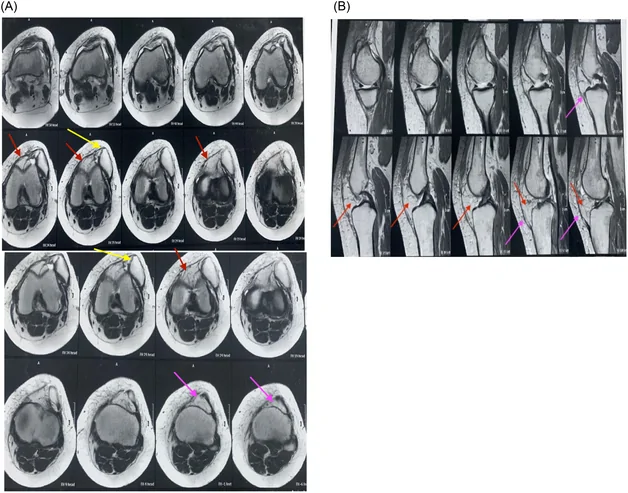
(A) Preoperative axial view MRI of the left knee. Yellow arrows, showing laterally dislocated patella. Red arrows, showing hypertrophied patellar fat pad; pink arrows, showing intact patellar tendon. (B) Preoperative sagittal view MRI of the left knee. Red arrows, showing hypertrophied patellar fat pad; pink arrows, showing intact patellar tendon. (MRI, magnetic resonance imaging.)
